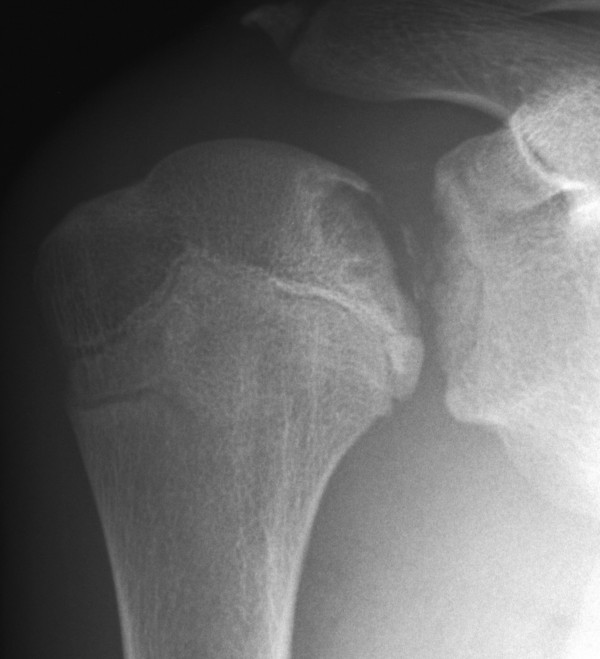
An anteroposterior plain radiograph (2007-5-25) shows the lesion became larger and a sclerotic band appeared around the lesion.
